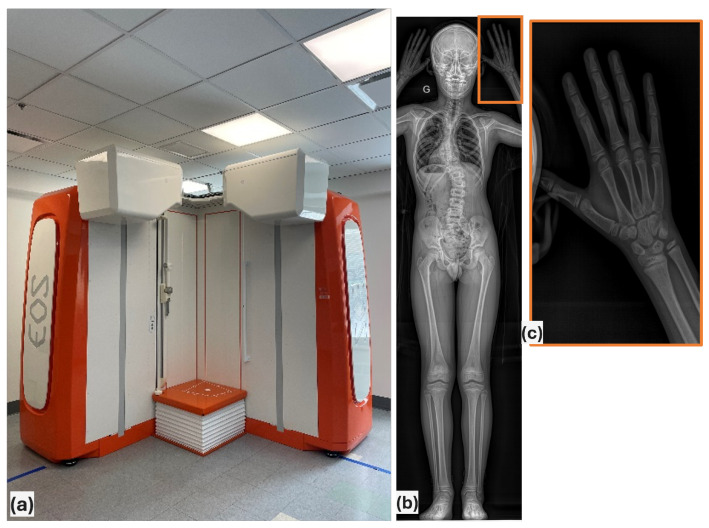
(a) EOS imaging system Pancorporal, (b) Radiograph of AIS patients, (c) Matching hand/wrist images [4].
